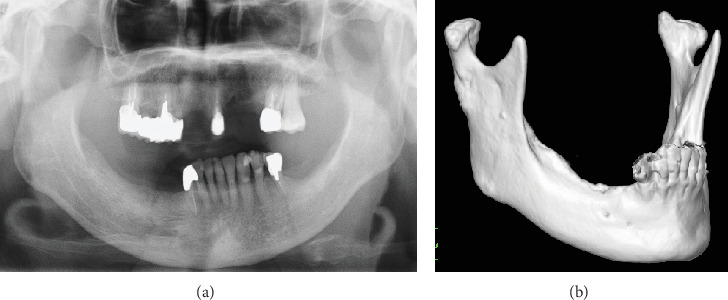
(a) Orthopantomography and (b) three-dimensional computed tomography image at 2 years postoperatively.
